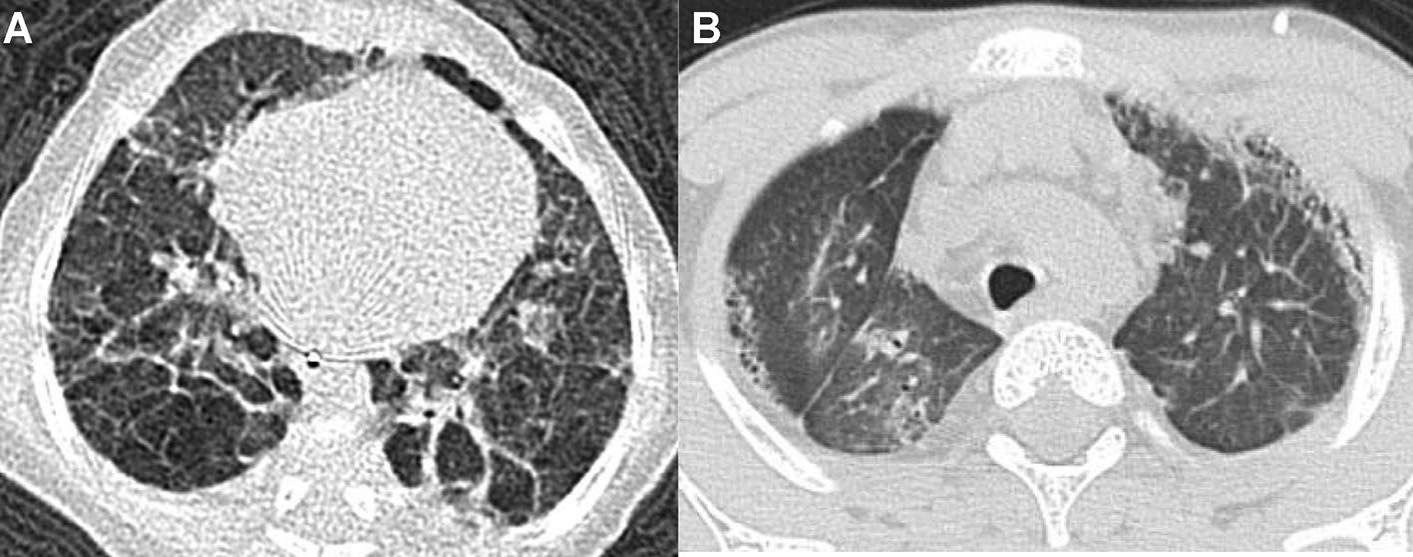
Alveolar growth abnormality due to broncho-pulmonary dysplasia (BPD) or chronic lung disease of prematurity (2 cases). A An infant prematurely born due to chorioamnionitis. CT at age 4 months showed inhomogeneous hyperlucency in both lungs associated with distorted bronchovascular shadows. B An 8-year-old boy with a history of chronic lung disease of prematurity. CT showed numerous subpleural microcysts that indicate a high likelihood of alveolar growth abnormality
